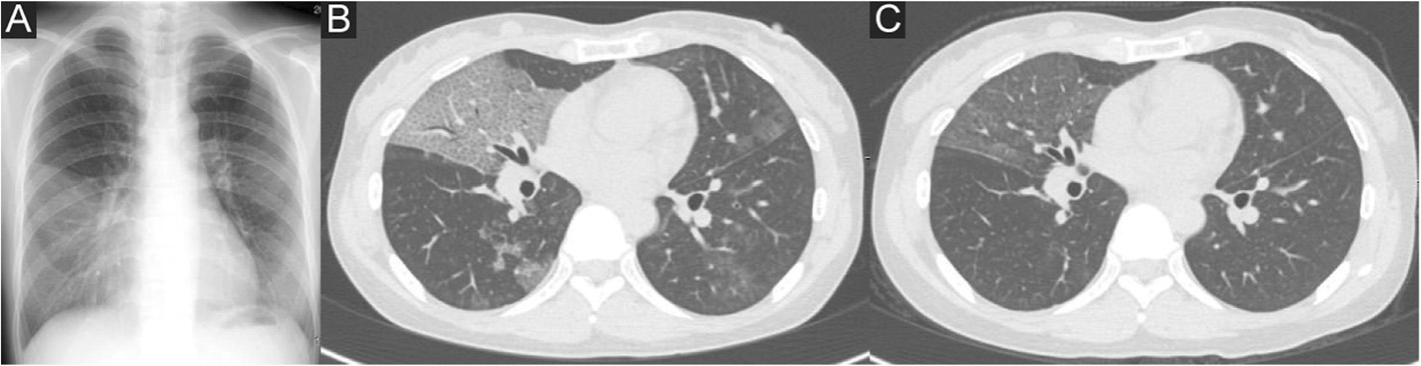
A chest roentgenogram and computed tomography images of a 38-year-old female with lipoid pneumonia. Infiltrative shadows were noted in the bilateral lower lung fields on admission (a). Chest CT revealed ground-glass opacities with interlobular septal thickening (crazy-paving pattern) and partial infiltration in the bilateral lower lung fields (b). The shadows improved 3 months later (c)
